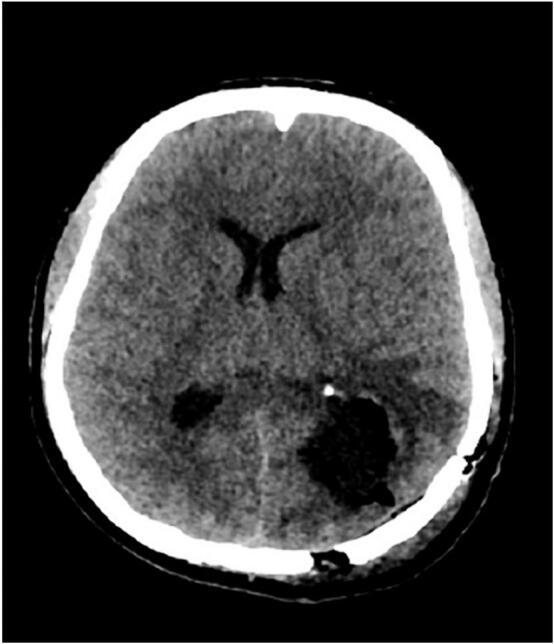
Follow-up computed tomography scan confirming complete removal of the lesion.
